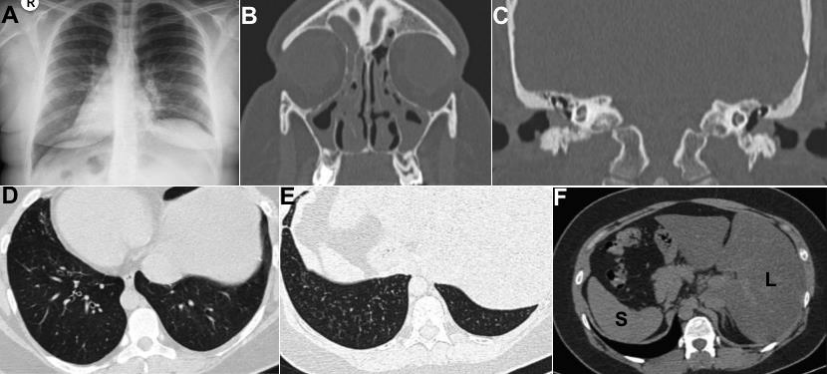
Kartagener’s syndrome: A) chest radiography with dextrocardia; B,C) face CT with mucosal thickening and material with soft tissue density in the paranasal sinuses, mastoid cells and in the middle and external ear cavities; D,E) axial chest CT showing bronchiectasis and centrilobular nodules (tree-in-bud sign); F) axial CT image showing liver (L) on the left and spleen (S) on the right
